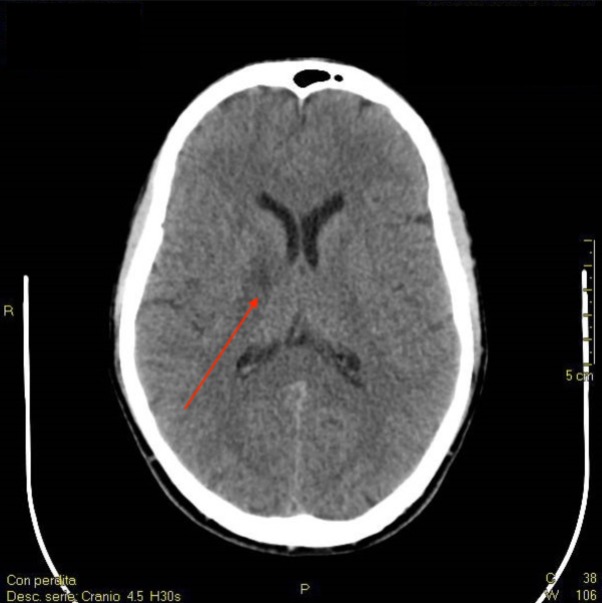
Brain CT scan showing a slight hypodense area in the right internal capsula (red arrow).
